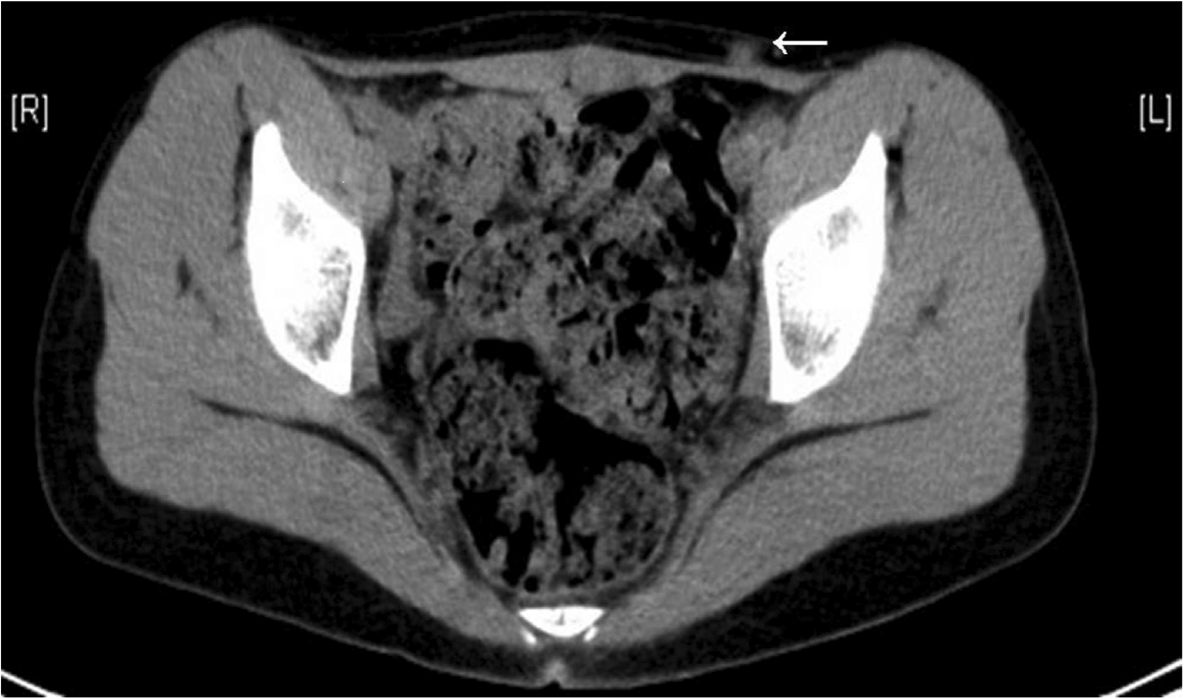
Axial computed tomographic scan without contrast agent displaying left inguinal soft tissue. Suspicious hyperdense, irregularly shaped lesion (*arrow*) with a diameter of approximately 8mm in the left inguinal soft tissue
